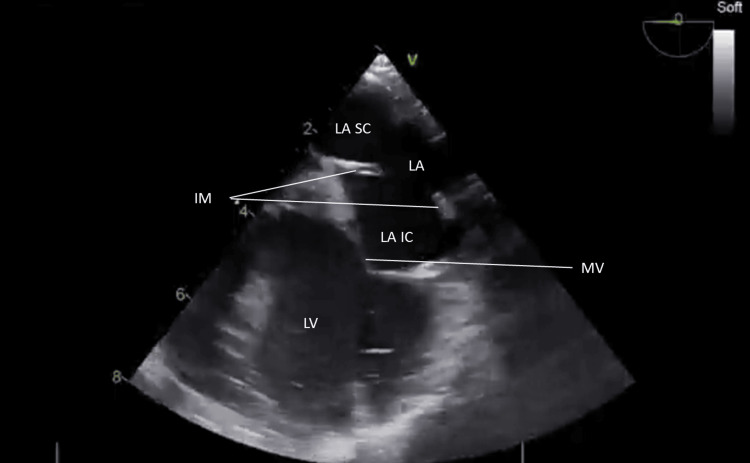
Transesophageal echocardiography at 0° shows an IM in the left atrium (LA), dividing it into superior (LA SC) and inferior compartments (LA IC), consistent with an incomplete, non-obstructive cor triatriatum sinister MV: Mitral valve; LV: Left ventricle; IM: incomplete membrane.
